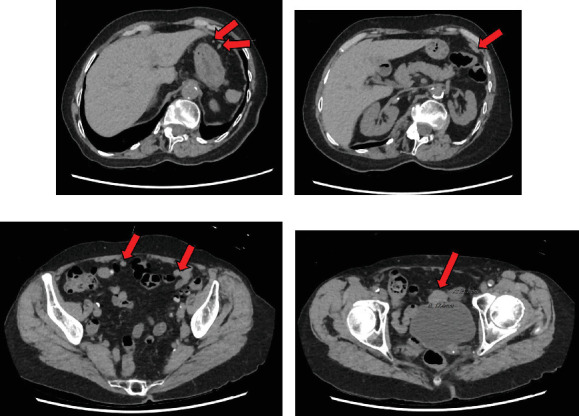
Case 2 radiographic findings. CT abdomen and pelvis (coronal view) showed multiple soft tissue lesions throughout the (a, b) omentum and (c, d) pelvic peritoneum, the largest located anterior to the bladder and measuring 2.5 cm.
